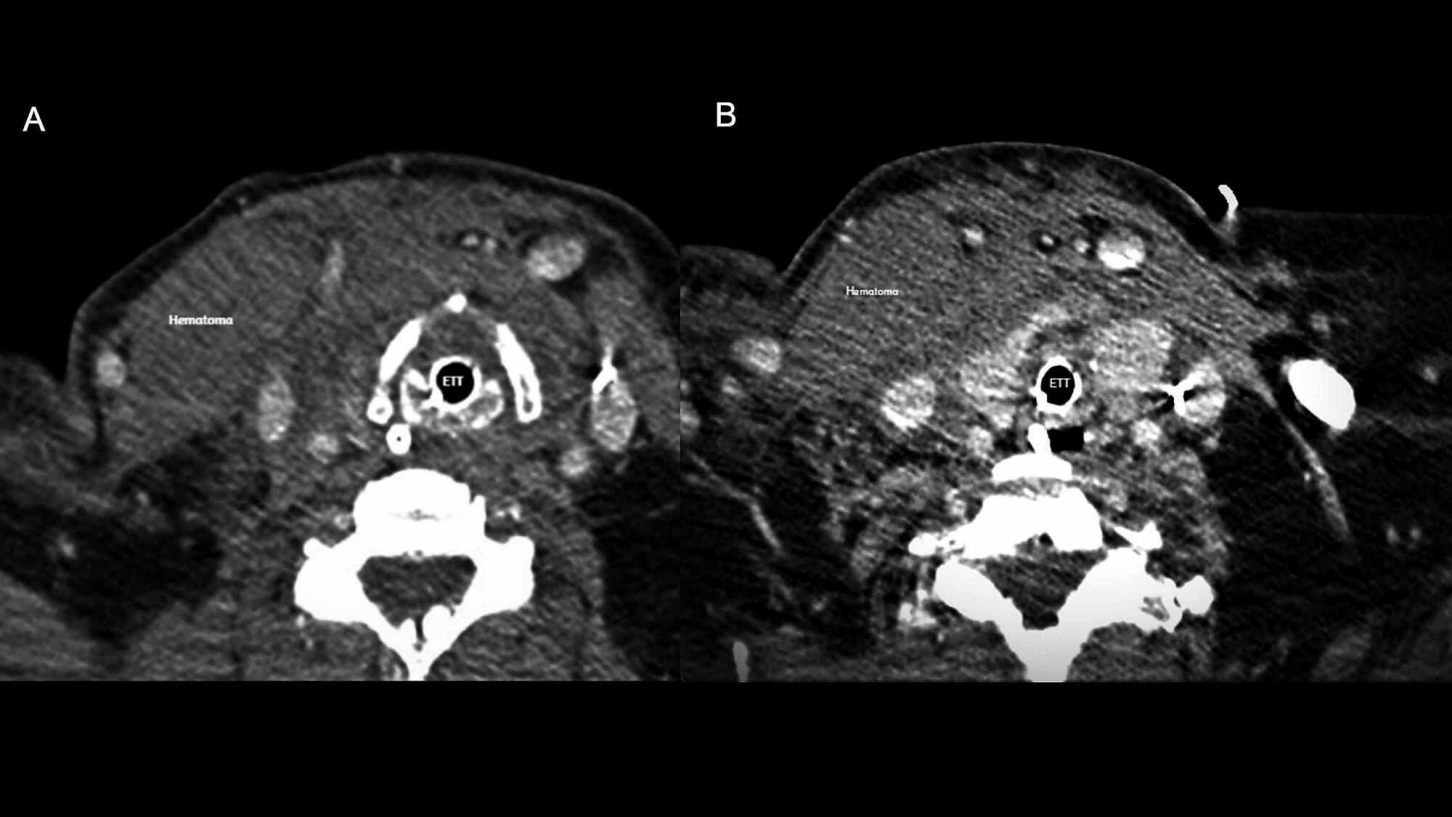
CT scan of the neck with IV contrast showing compressive hematoma of the airway progressing as we go from A to B. ETT stands for endotracheal tube
